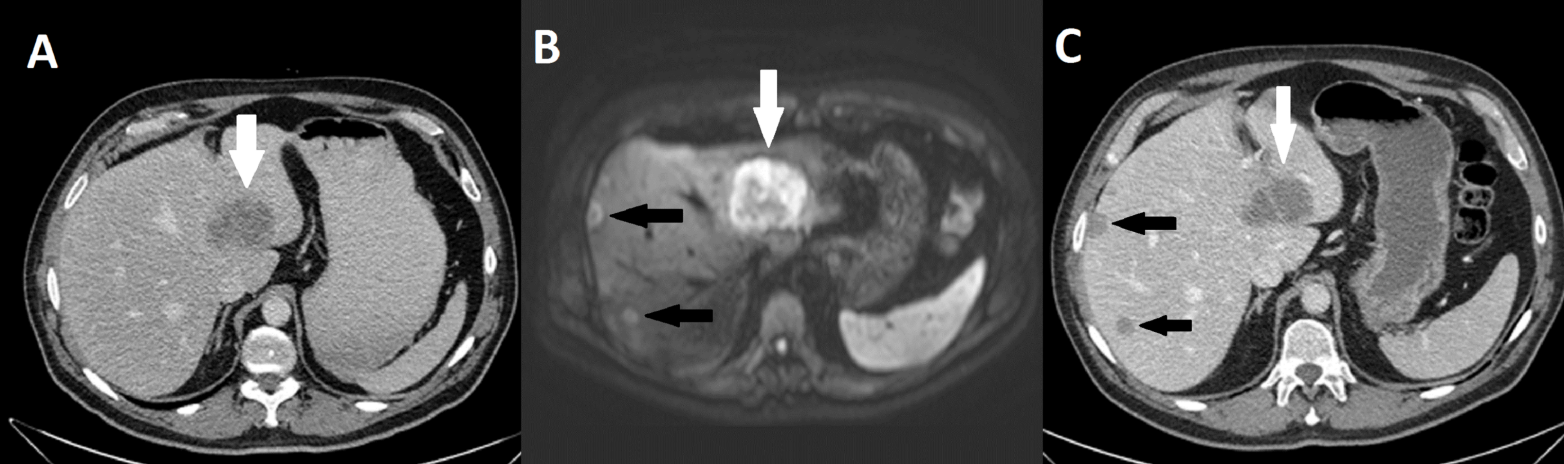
Missed liver metastasis segment 7 & 8 on CT and diffusion weighted MR. (A) Initial CT, (B) Diffusion weighted MR, (C) CT in venous phase. White arrow: Segment 4 metastasis. Black arrow: Two metastases missed on primary CT.
